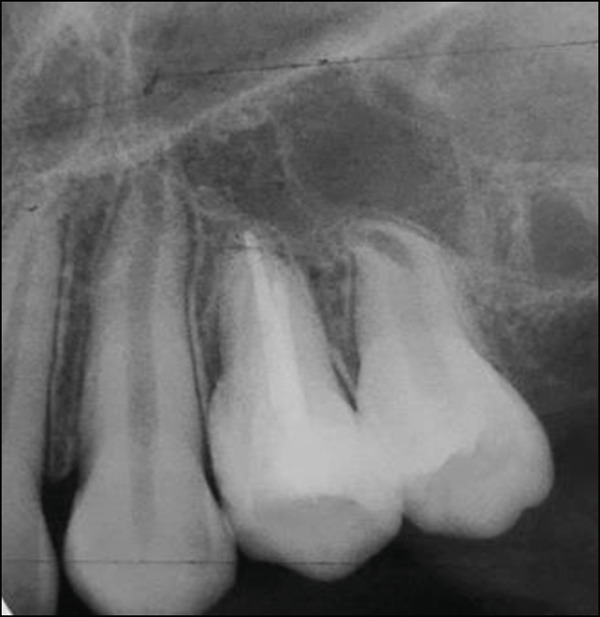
Postoperative periapical radiograph of 24.
